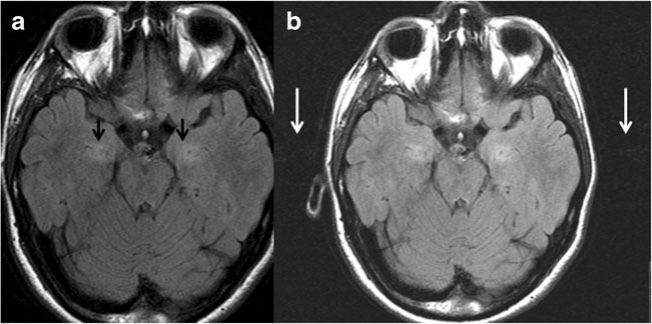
A 25-year-old man referred to MRI due to headaches. On axial FLAIR images, there is suspicious hyperintensity in the medial temporal lobes without atrophy or mass effect (*black arrows*). The abnormality was not enhancing and was not clearly seen on sagittal T2 weighted images. By using a wider window, repetitive ghosting is seen outside the anatomy along the phase encoding direction, compatible with pulsation artifact
